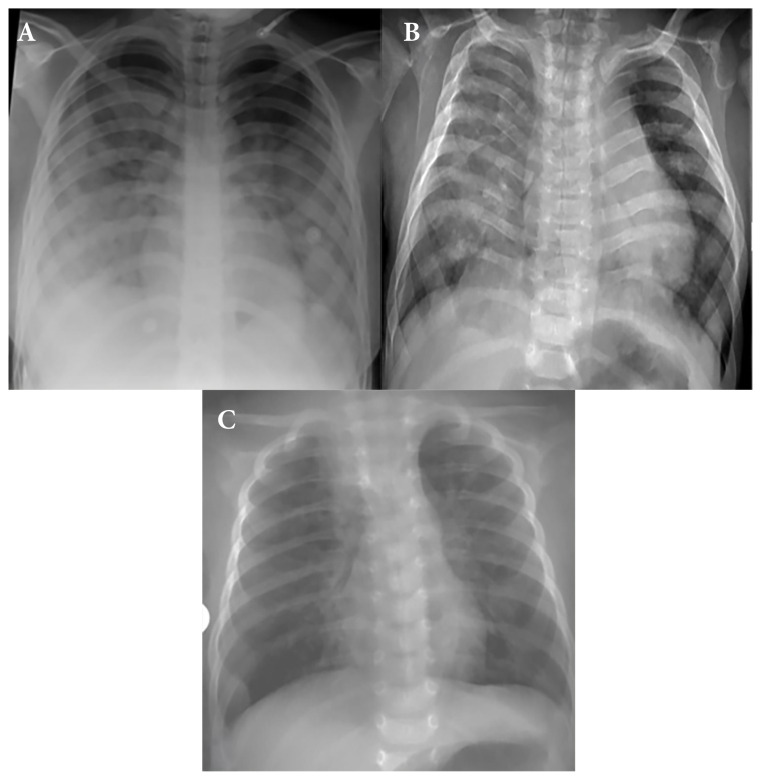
A) Patient 221: 14-year-old girl with autoimmune polyglandular syndrome. Bilateral mainly peripheral ground glass opacities was seen at middle and lower zones, on chest X-ray, diagnosed as COVID-19, B) Patient 40: 1-year-old boy with Schinzel Giedion syndrome. Multifocal infiltrations were seen on X-ray, predominantly on the right side. Hyperinflation was prominent especially in the left lung. Rhinovirus was detected at RVP tests, C) Patient 171: 1-month-old immigrant boy who had a history of contact with COVID-19 positive patient. Chest X-ray showed bilateral peribronchial opacities and overinflation. Diagnosis of RSV pneumonia was made after RVP tests.
